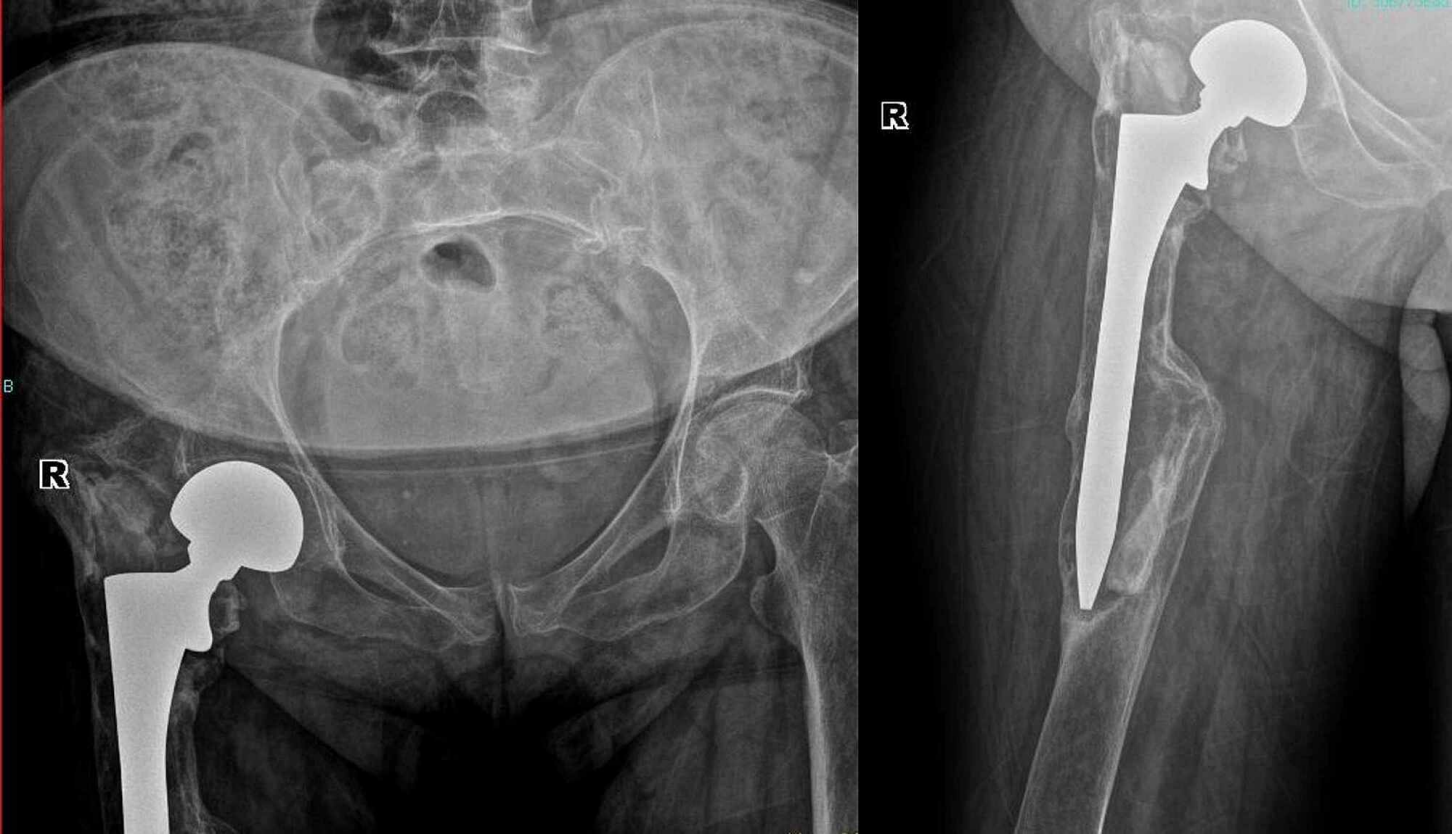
The preoperative radiographs of the patient with loosening of the right hip prosthesis.
